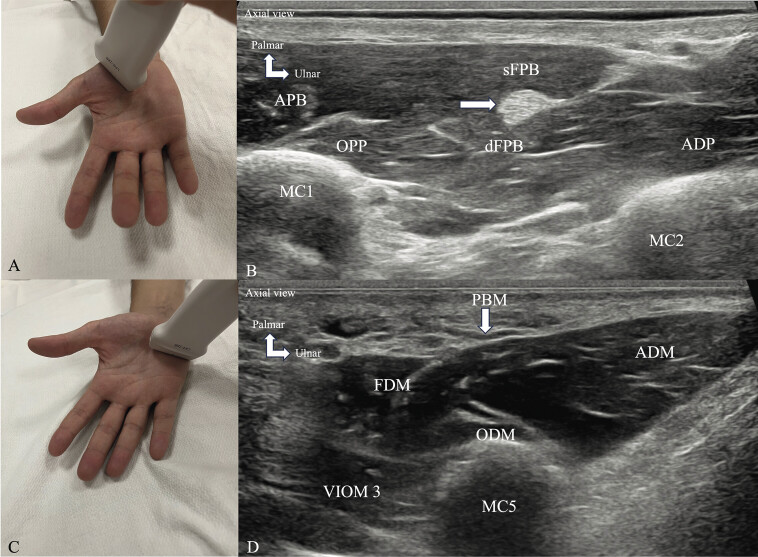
Normal ultrasound appearance of thenar muscles (
